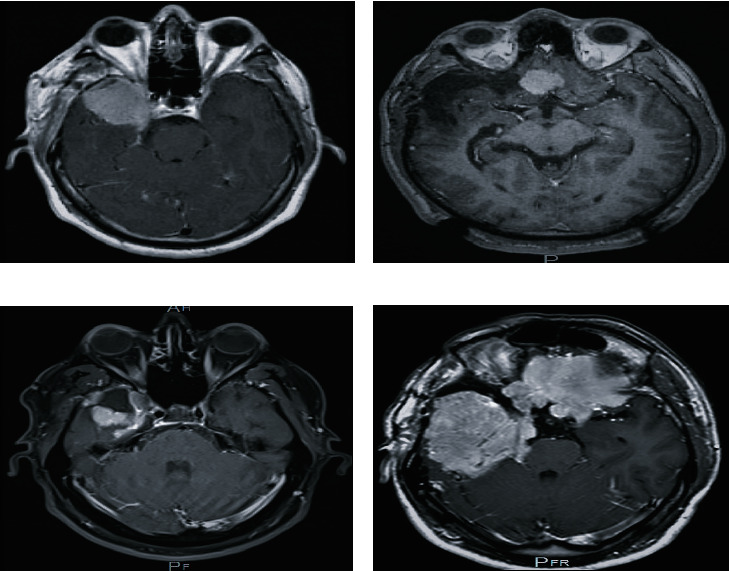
Neuroimaging–MRI ((a–d) with contrast enhancement) shows (a) sphenoid wing meningioma. (b) Frontobasal tumor projecting towards the right side (pre-SRS). (c) Tumor residual occupying the posterior aspect of the sphenoid wing and parasellar region (post-SRS). (d) Tumor occupies all the right middle cranial fossa extending to the cavernous sinuses, sella, and suprasellar regions as well as right prepontine and CP angle. It is also spread to the ipsilateral right superior orbital fissure and a lesser extent optic canal. The second component within the left anterior cranial fossa crossing the midline to the contralateral side and posteriorly to the left sphenoid ridge (post-SRS).
